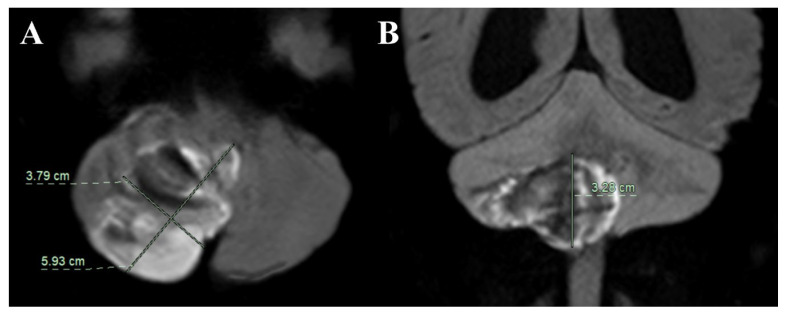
Measurement of infarct volume on diffusion-weighted imaging. (A) Axial DWI showing the maximum lesion diameter (5.93 × 3.79 cm) in the right cerebellar hemisphere. (B) Coronal DWI image demonstrating the craniocaudal extent (3.28 cm). The infarct volume was estimated to be approximately 38.8 mL using a simplified ellipsoid formula.
